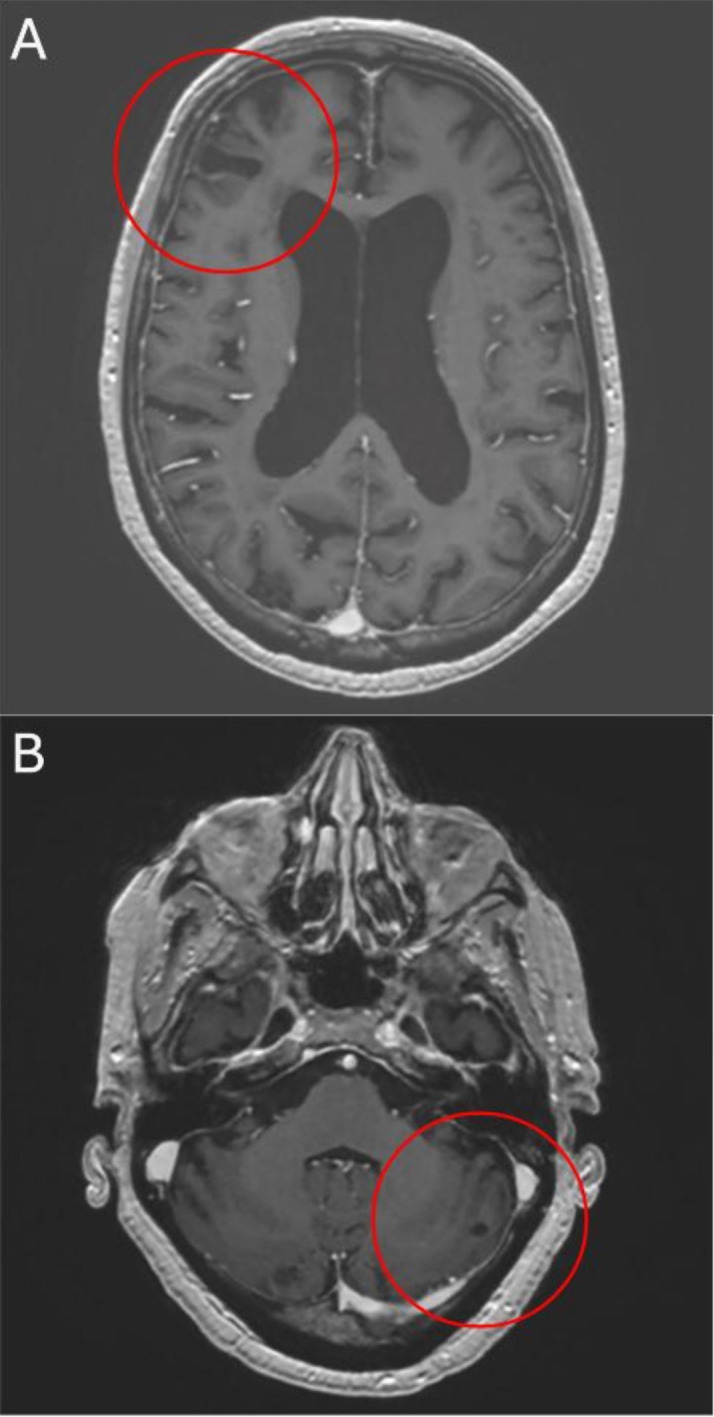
After 11 months of treatment axial T1-weighted MRI shows further reduction of right frontal (A) and left cerebellar (B) lesions.
